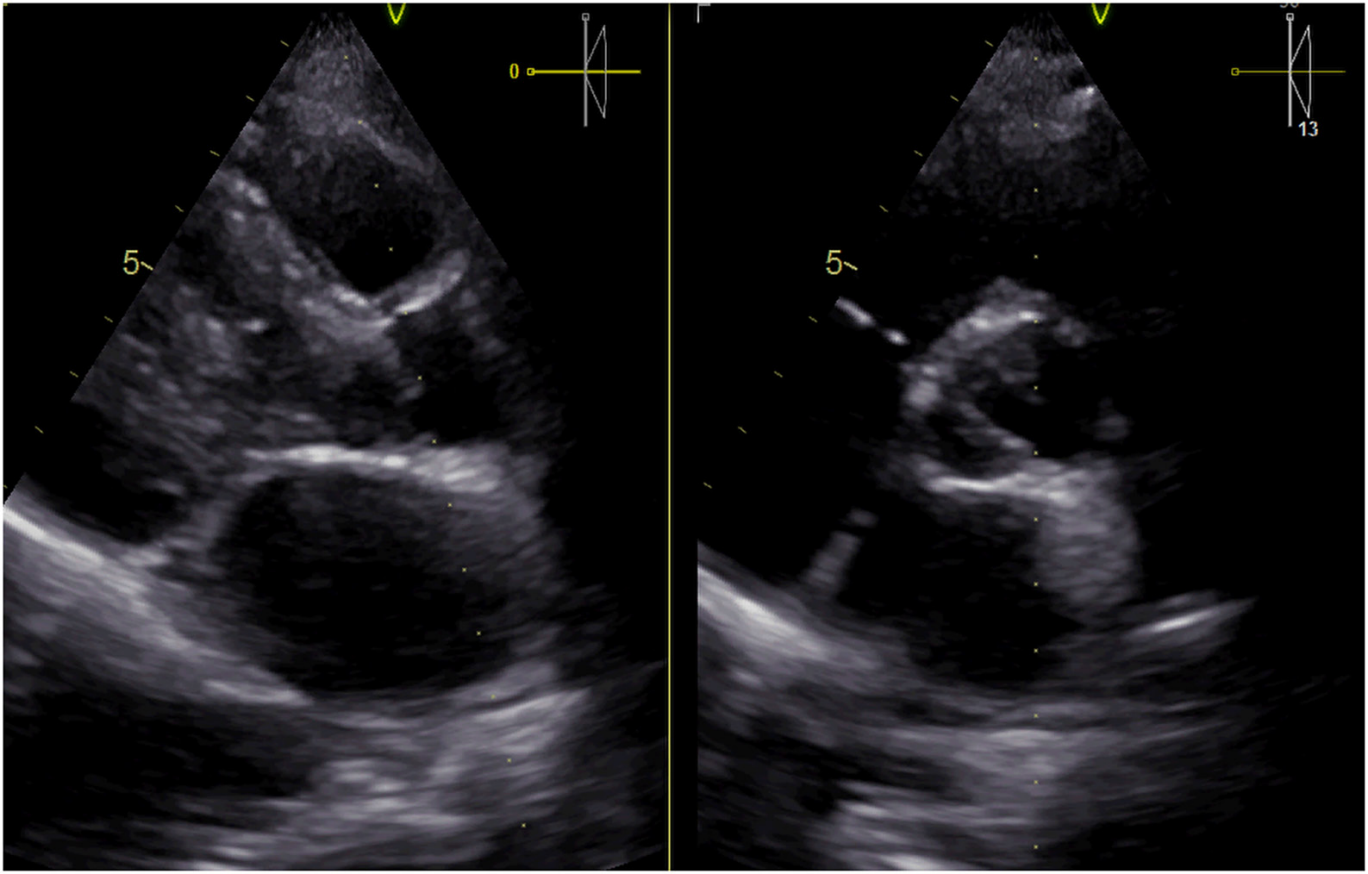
Aortic valve area planimetry from transesophageal echocardiography.
